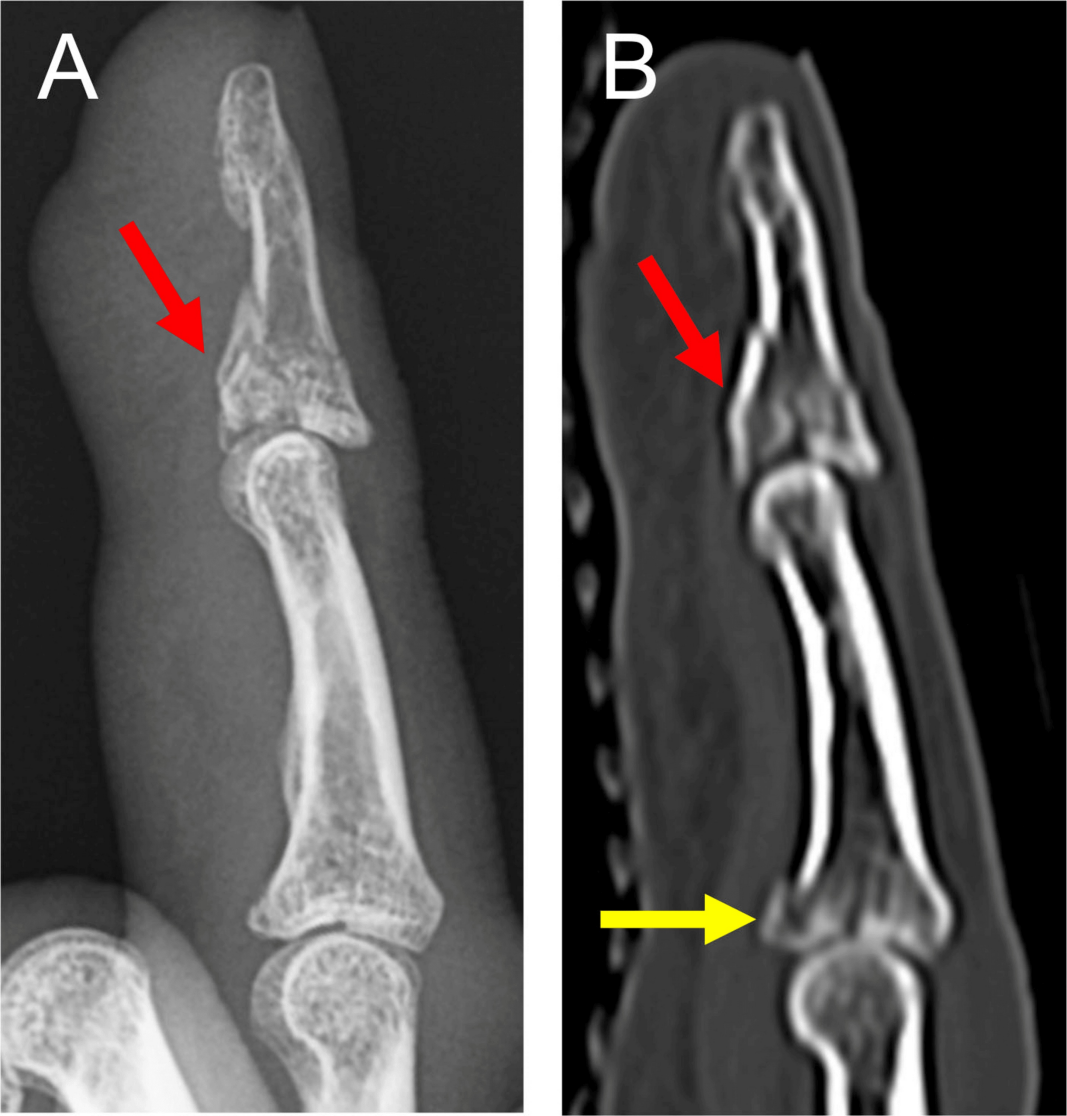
Preoperative radiograph and CT scan of Patient 3 Radiograph and CT scan showing double dorsal fracture-dislocation of the DIP and PIP joints of the right ring finger. The lateral view of the radiograph (A) and sagittal view of the CT scan (B) show a split-depression type fracture of the distal phalangeal base (red arrows). Dorsal subluxation of the PIP joint with displaced and impacted volar fragment of the middle phalangeal base (yellow arrow) is seen. DIP: distal interphalangeal; PIP: proximal interphalangeal
